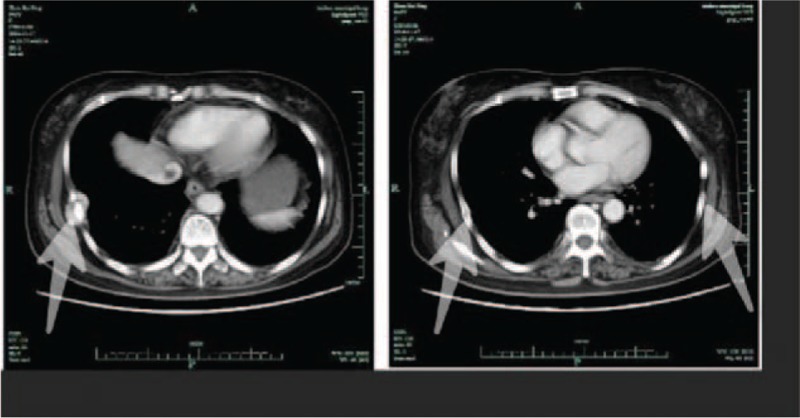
Chest computed tomography (CT) suggested multiple bilateral rib fractures (indicated by white arrow), destruction of the right side of the 7th, 8th rib bone, and a local soft tissue mass.
